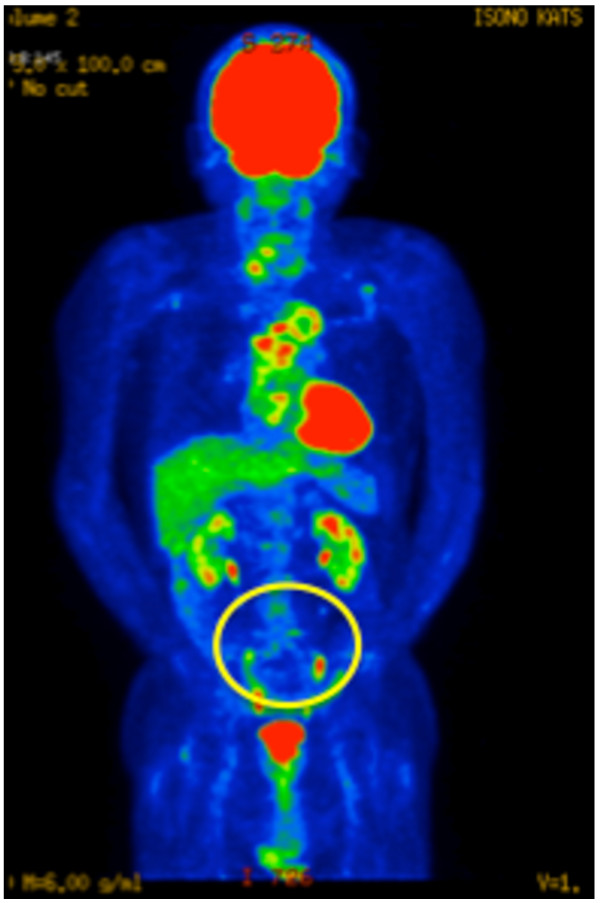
^18^ F-FDG-PET/CT in Case 1, 18 months after treatment for pyogenic spondylitis. It showed resolution of the abnormal accumulation of ^18^ F-FDG in L5, while both SUVmax (6.43) and the distribution pattern of ^18^ F-FDG around the aortic graft were unchanged. *Circle*; Abnormal accumulation of ^18^ F-FDG in L5 has disappeared.
